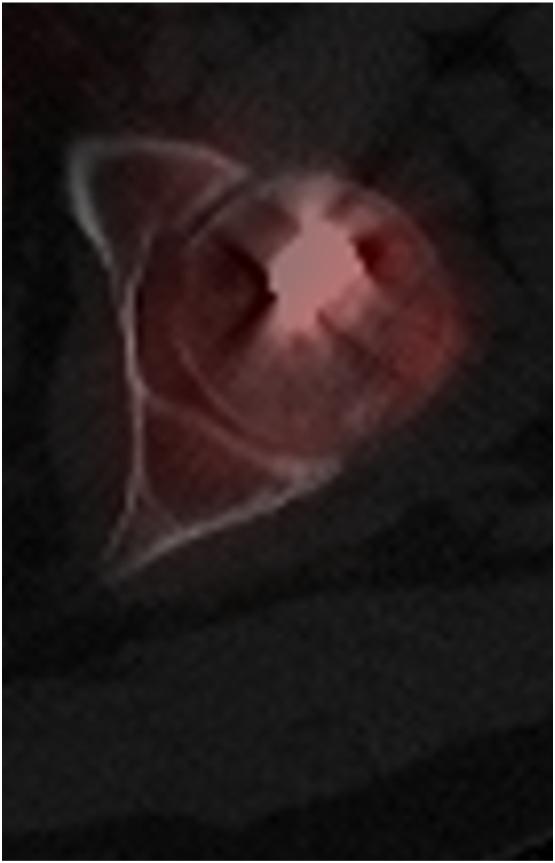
PET-CT of the left hip showing persistent femoral head vascularity.
